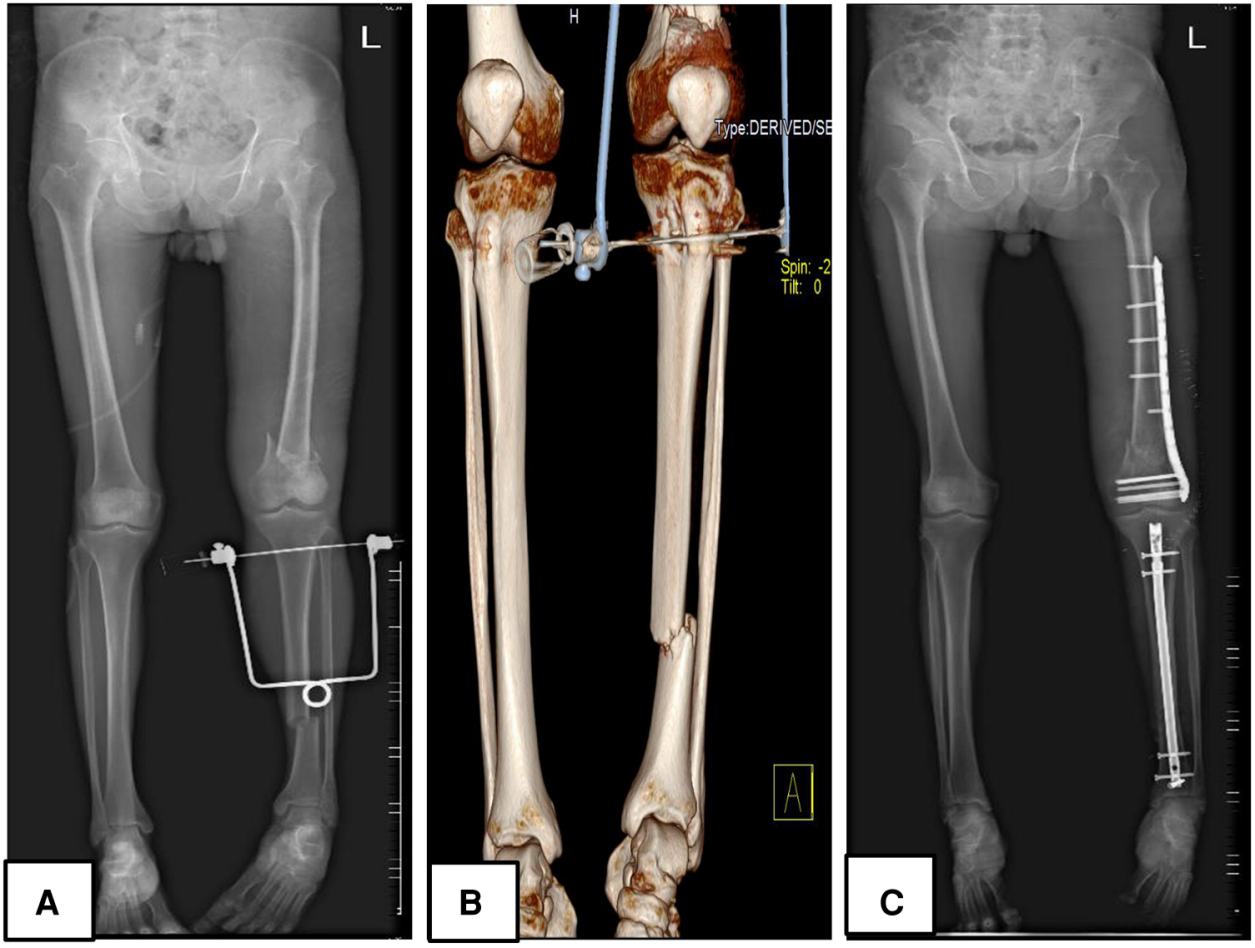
A 59 years-old male patient (patient number 33) who sustained a Fraser type IIB floating knee following a road traffic accident managed surgically; (A,B) represent preoperative plain radiograph and 3D reconstruction CT images respectively; (C) displays a postoperative plain radiograph of the lower limb, the femur was managed with a plate while the tibia was treated with an intramedullary nail.
